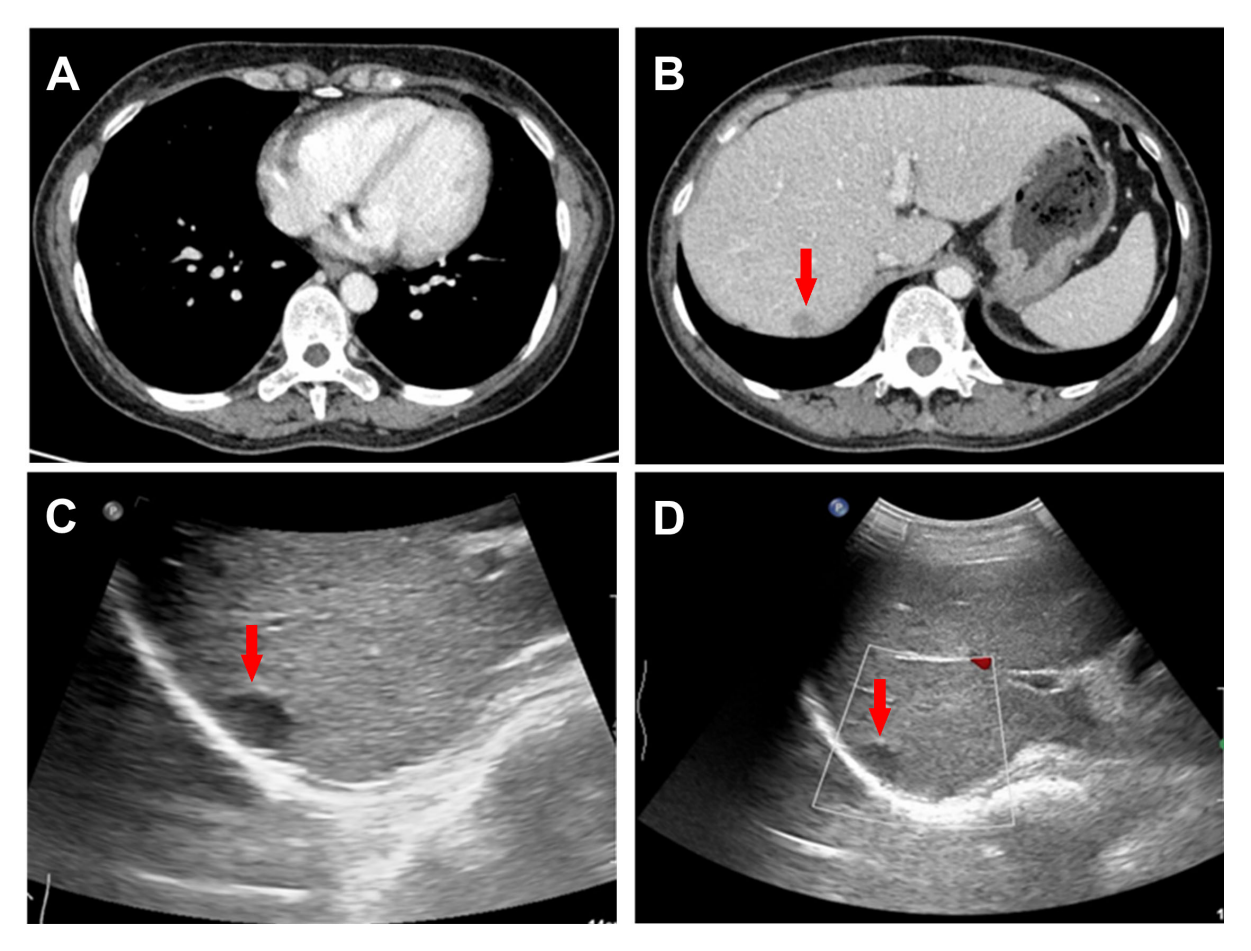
CT presented thickened interlobular septa in both lungs (A) and a 1.1cm hypodense nodule in the right upper posterior lobe of the liver (B). Conventional ultrasound revealed a 1.4 × 1.1cm hypoechoic nodule in the upper segment of the right posterior lobe of the liver (C, D).
